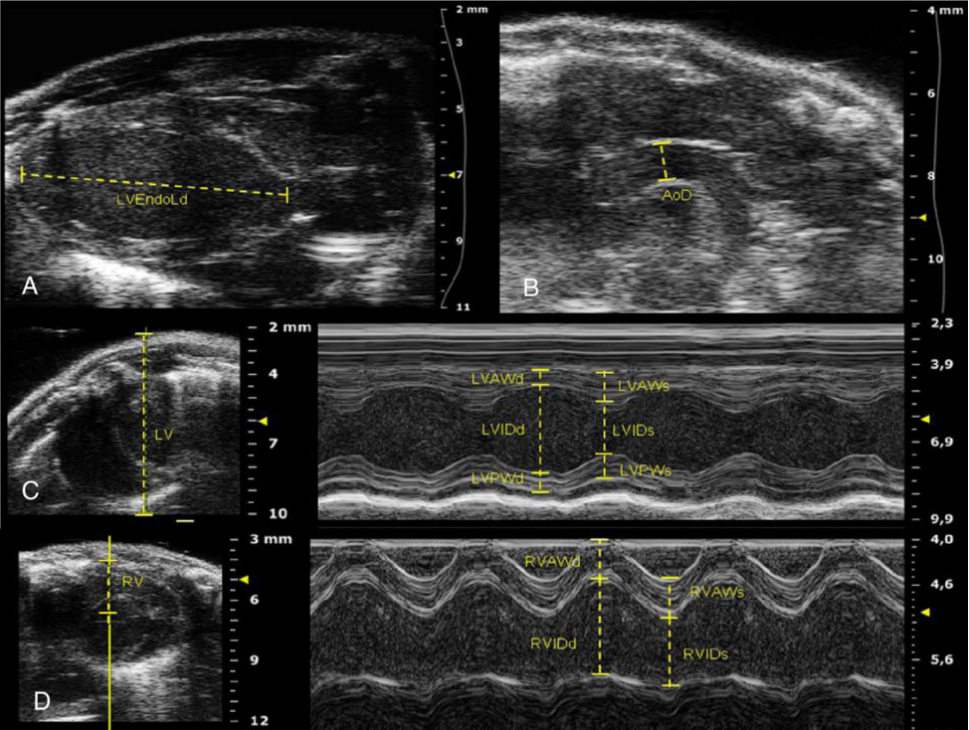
Representative B-mode and M-mode echocardiographic images and measurements. (A) Parasternal long axis view of left ventricle (LV) in B-mode. (B) Aortic arch view in B-mode. (C) Short axis view in 2D (left panel) and M-mode tracing (right panel) of the LV, at the level of papillary muscle. (D) Right parasternal long axis view in 2D (left panel) and M-mode tracing (right panel) of the righ ventricle (RV). LVEndoL = Left ventricle endocardial length. AoD = Ascending aorta diameter. LVAW = Left ventricle anterior wall. LVID = Left ventricle internal diameter. LVPW = Left ventricle posterior wall. RVAW = Right ventricle anterior wall. RVID = Right ventricle internal diameter; -d = In diastole. -s = In systole.
